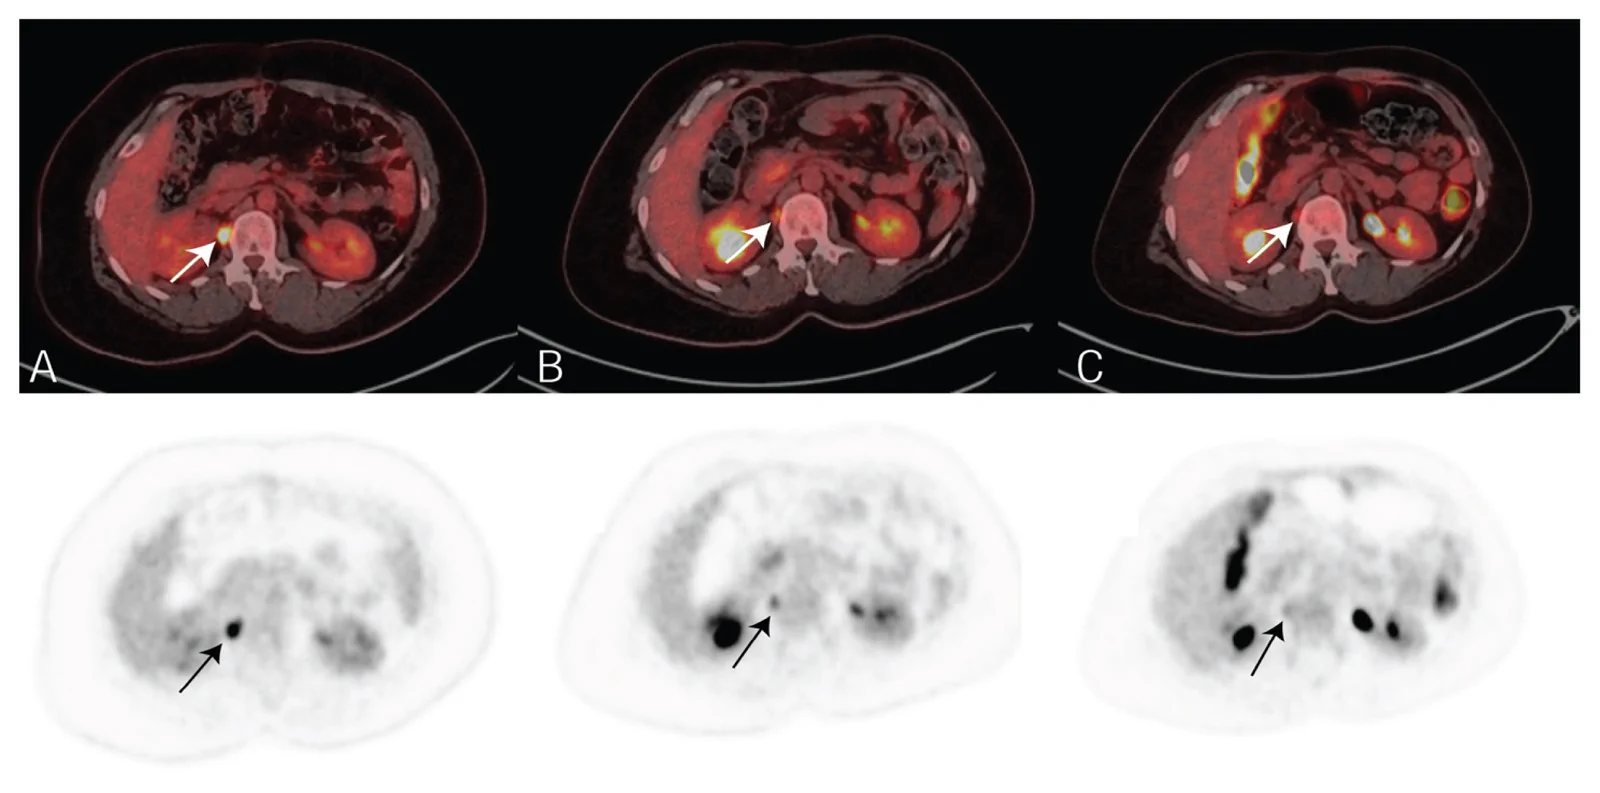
Endometrial carcinoma [18F] FDG-PET/CT at (A) base line, (B) post-6 cycles immune checkpoint inhibitor (ICI) and (C) post-12 cycles of ICI respectively demonstrating an avid right retrocrural lymph node with interval reduction in metabolic activity in the follow-up studies (arrows); in keeping with partial metabolic response.
